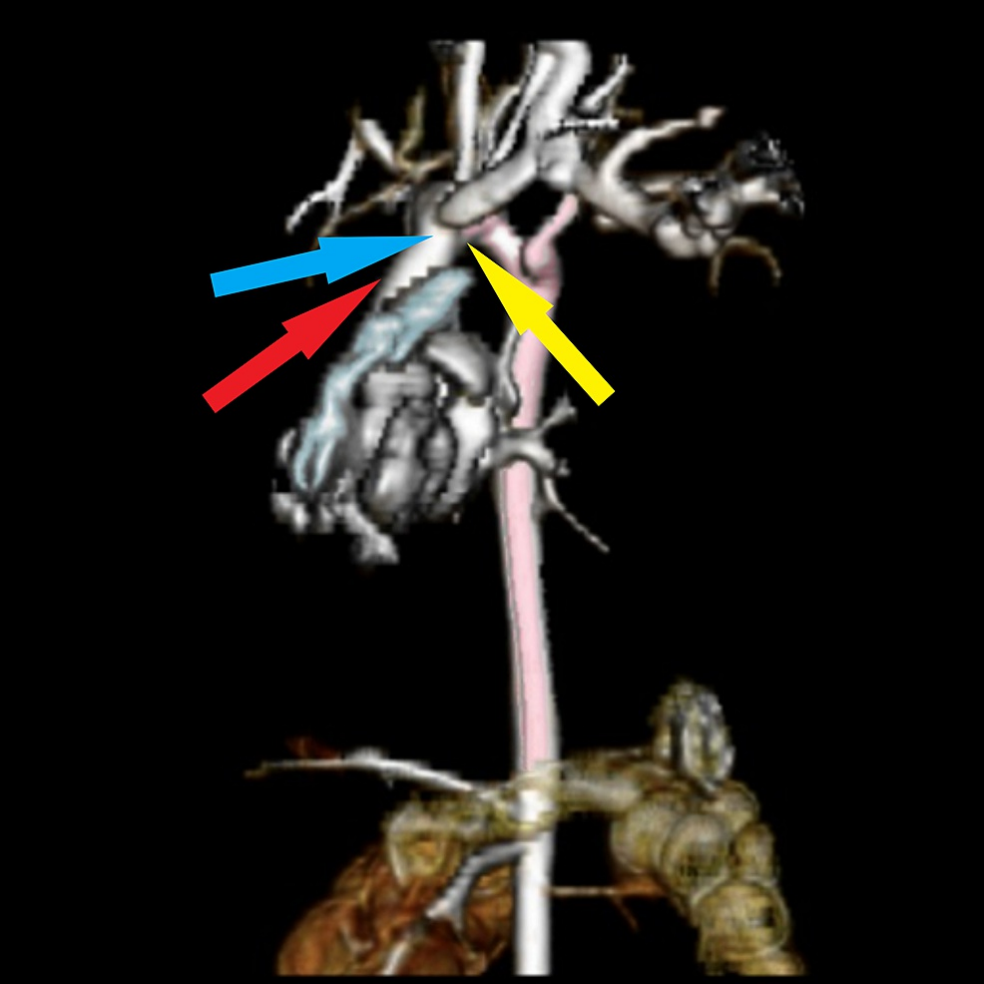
CT chest angiogram. Right-sided aortic arch with left aberrant subclavian artery with a Kommerell diverticulum. Red arrow: right-sided aortic arch; blue arrow: left aberrant subclavian artery; yellow arrow: Kommerell diverticulum.
